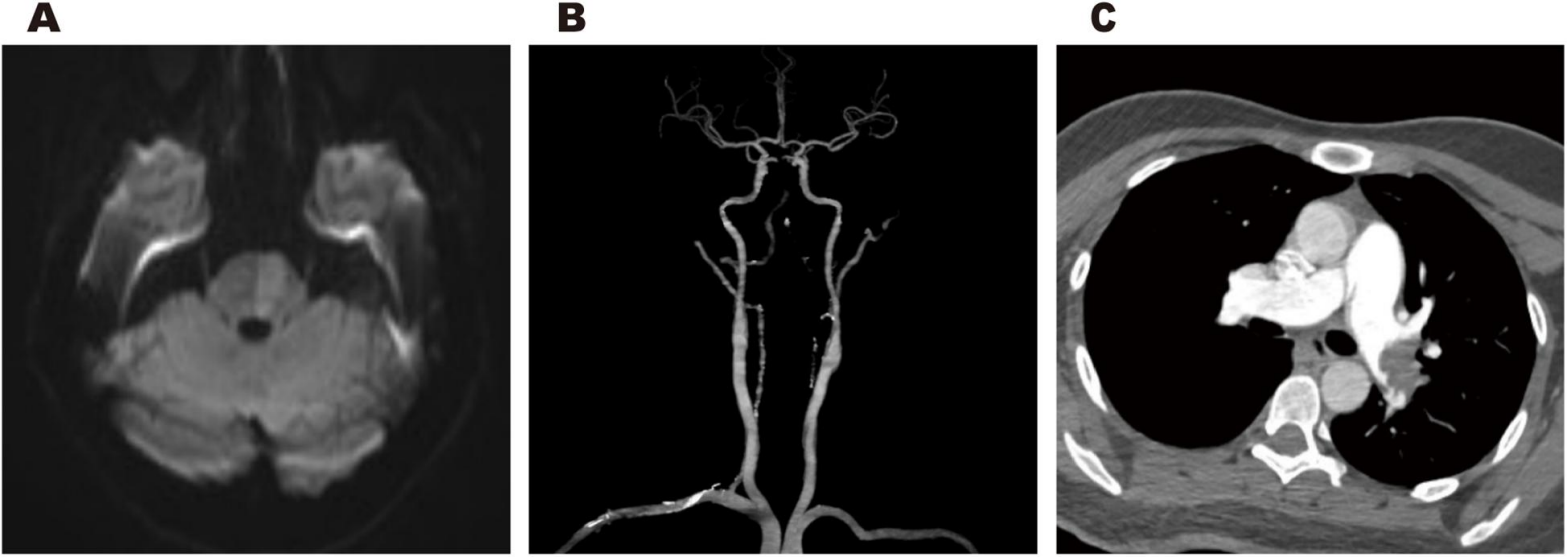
Cranial MRI revealed an acute pontine infarction (A). Head and neck CTA revealed basilar artery occlusion (B), and incidentally showed left pulmonary artery embolism (C).
